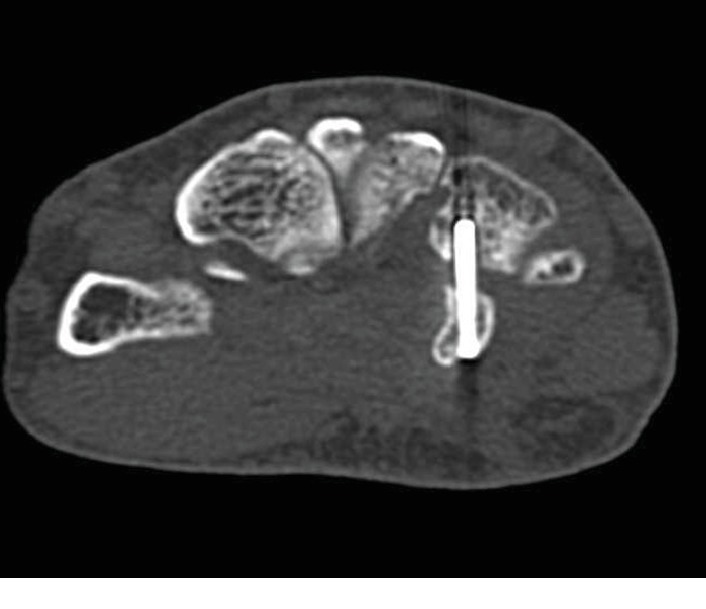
CT scan performed 3 months postoperatively showing the gap at the fracture site.
